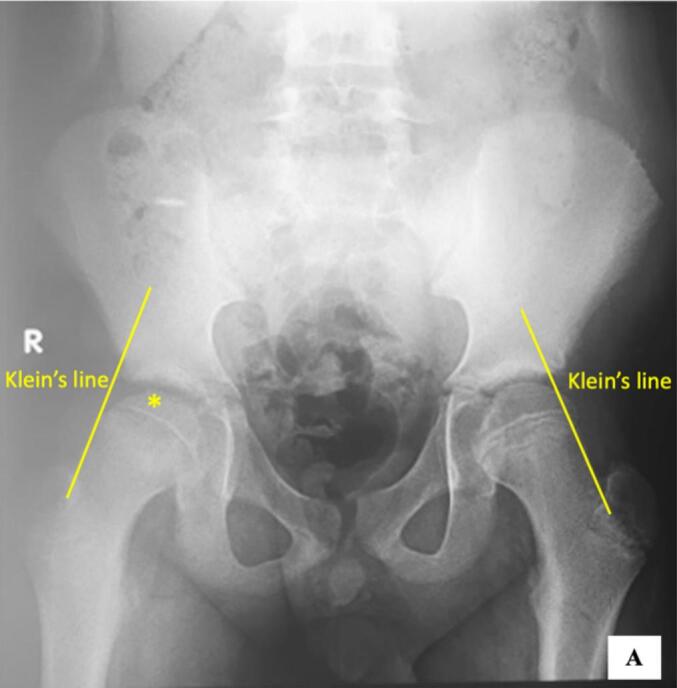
First anteroposterior pelvic plain radiograph. Depicted the AP pelvic radiograph after the first trauma. In the image, the asterix sign showed decreased epiphyseal height on the right hip compared to the left hip. Also on the right hip, Klein’s line did not cross the epiphysis.
